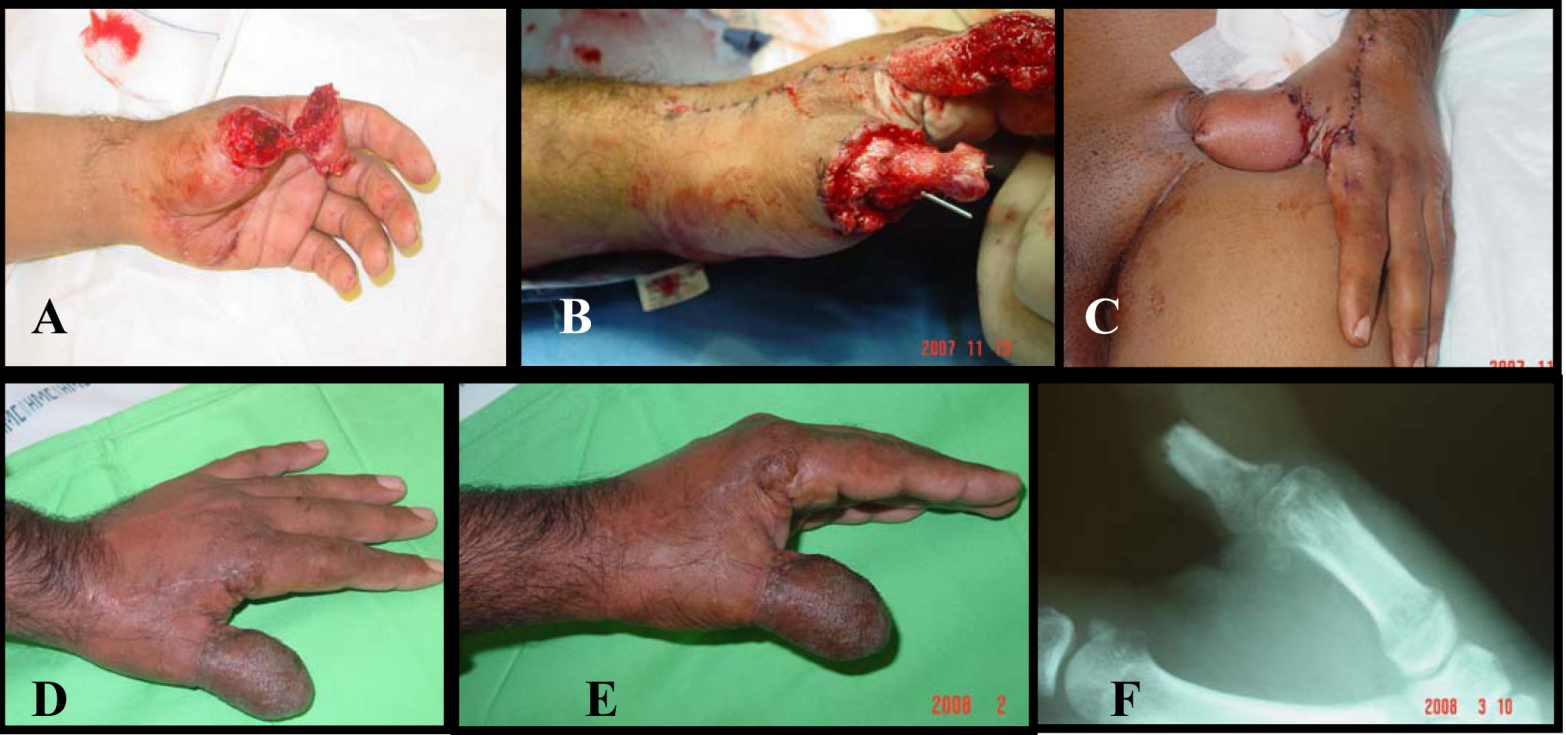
A: the amputation at two levels. B: Fixing the cortical bone graft. C: soft tissue cover by groin flap. D: division of the flap. E: final result. F: x-ray shows the bone healing.
